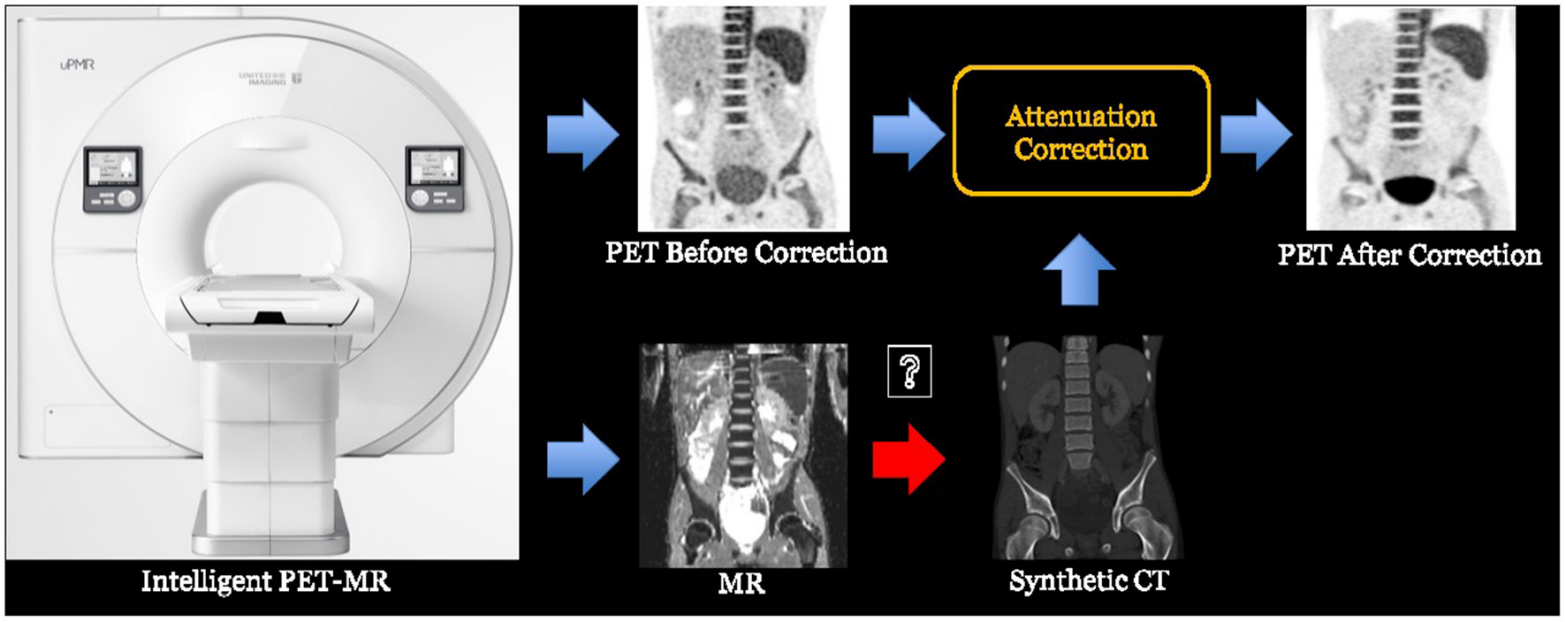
The process of PET-MR attenuation correction, where a synthetic CT image is obtained from the MR image to help the attenuation correction of PET image.
